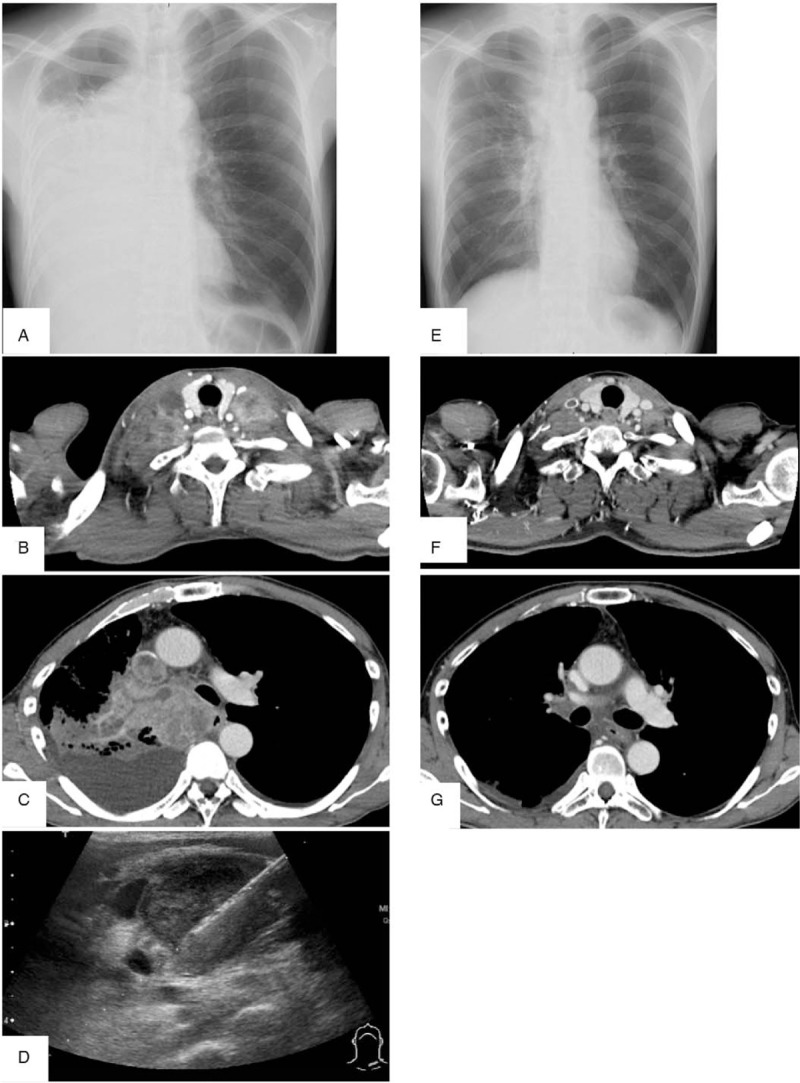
Chest radiography and computed tomography findings. A-C: before treatment initiation, E-G: after two cycles of combination therapy, D: percutaneous ultrasound appearance of needle biopsy of the left side of a cervical lymph node.
